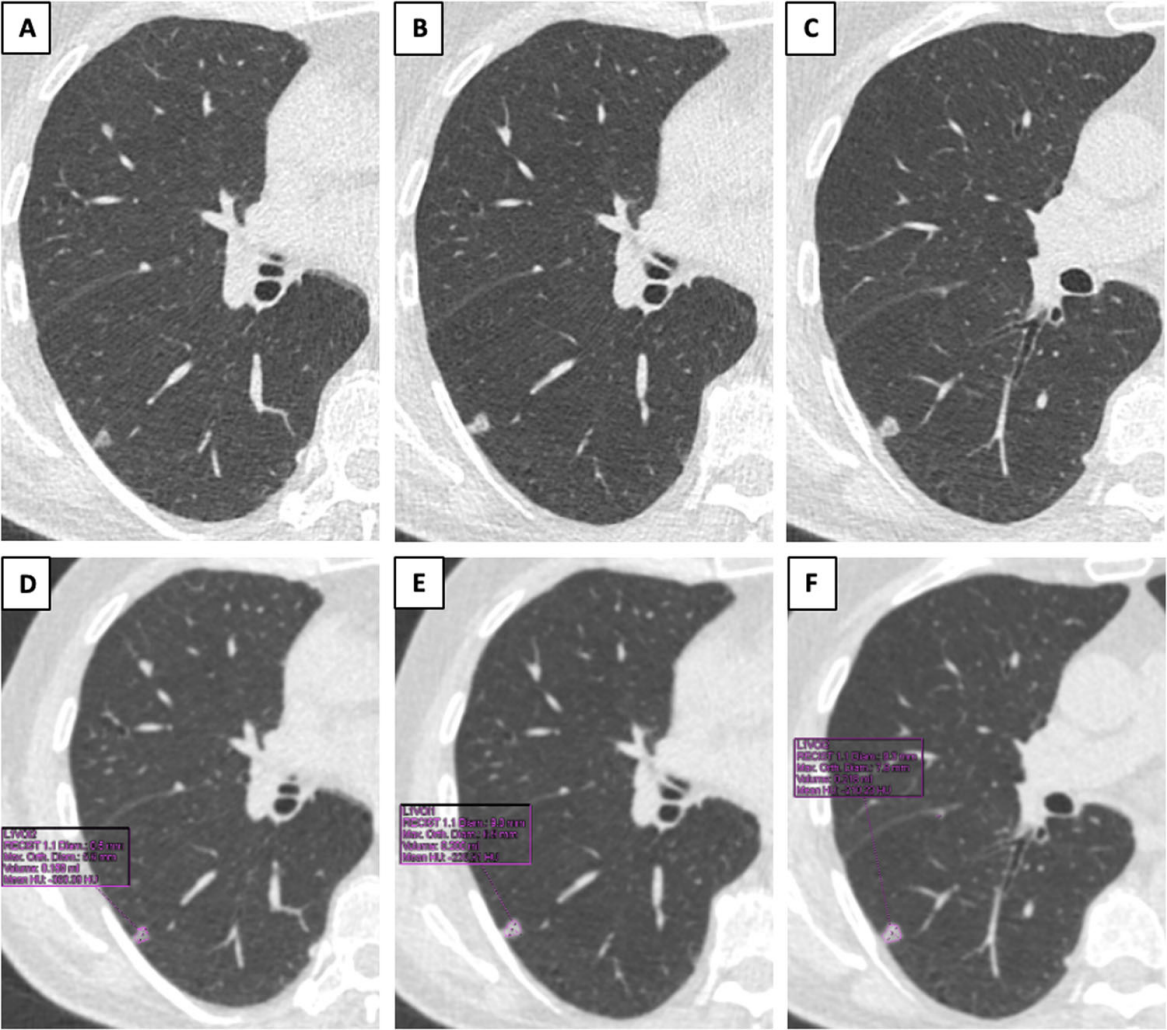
Example of a lung adenocarcinoma presenting as a non-solid nodule at baseline LCS low dose computed tomography (A), which developed a solid component at 12-months follow-up (B). A further increase in nodule size and density is shown in C. Nodule size and density for the three-time points are shown in D–F, respectively
